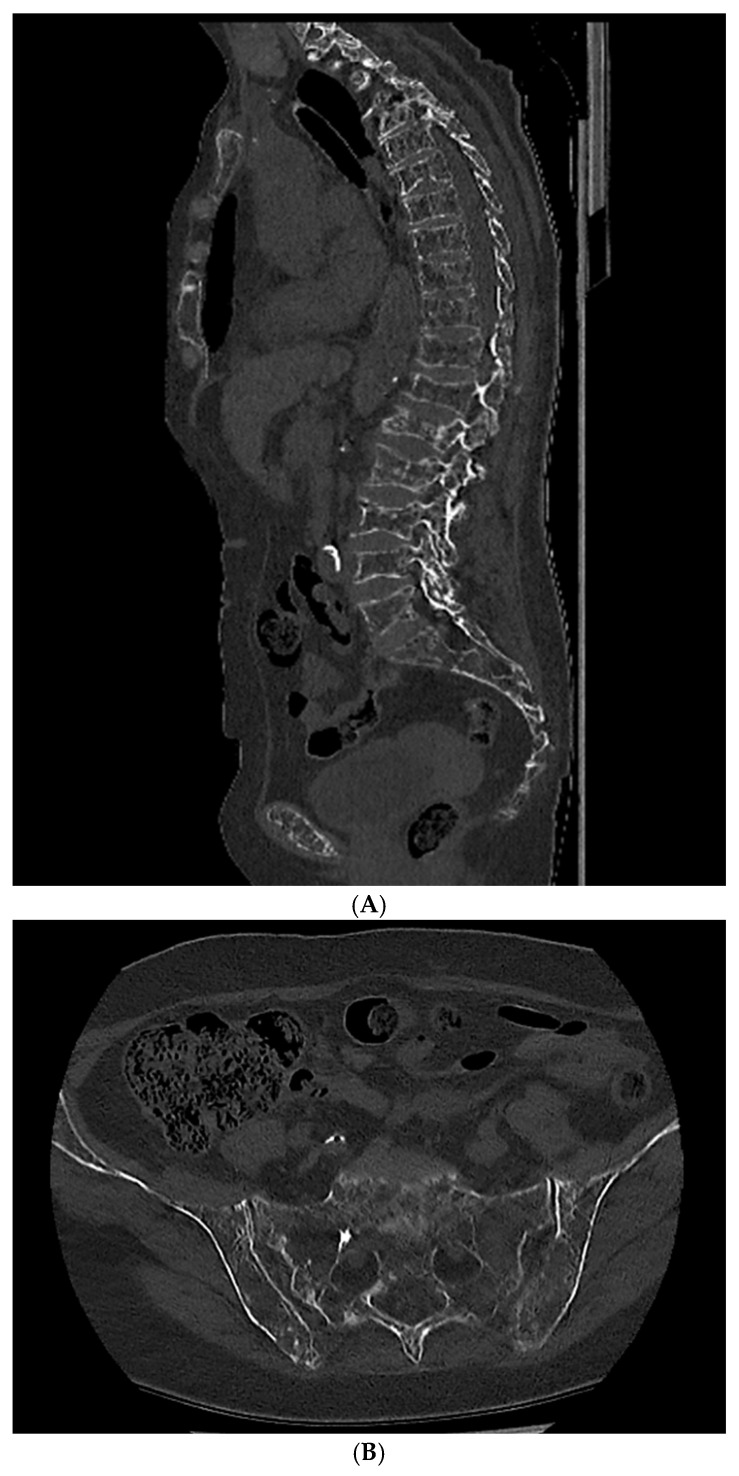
A 77-year-old woman with a diagnosis of multiple myeloma. (A)—Whole-body low-dose computed tomography demonstrates lytic lesions in (A) vertebral bodies and (B) sacral bone and iliac crest. Source: Radiological Diagnostics Center, The National Institute of Medicine of the Ministry of Interior and Administration, Warsaw, Poland.
